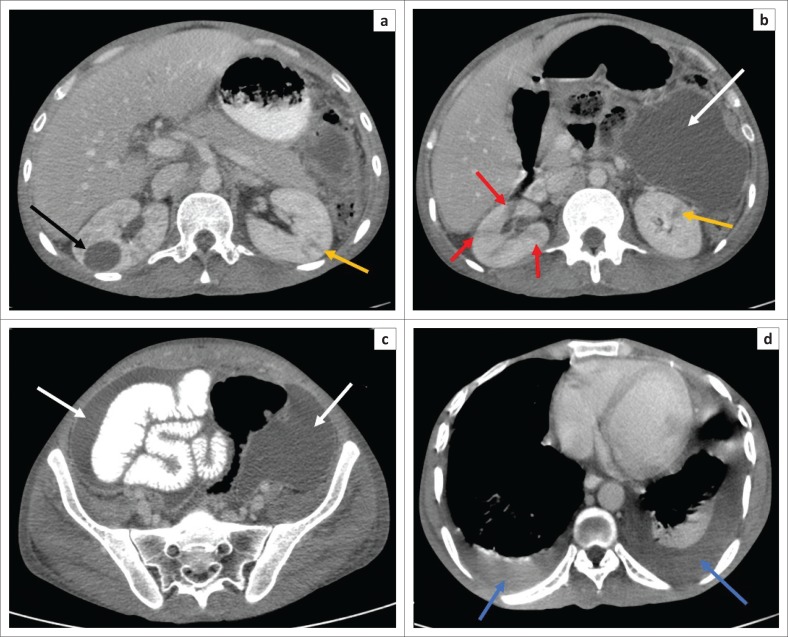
Post-contrast scan (venous phase) computed tomography (CT) abdomen: (a–d) Several small wedge-shaped areas of hypoattenuation in the right kidney in keeping with pyelonephritis (red arrow). Few similar areas are noted in the left kidney (yellow arrow). Large fluid-filled cavity in the right renal mid pole (black arrow). Moderate ascites with associated thickening and enhancement of the parietal peritoneum (white arrows) in keeping with peritonitis. There is also bilateral pleural effusion with associated relaxation atelectasis in the posterior left lower lobe (blue arrows).
